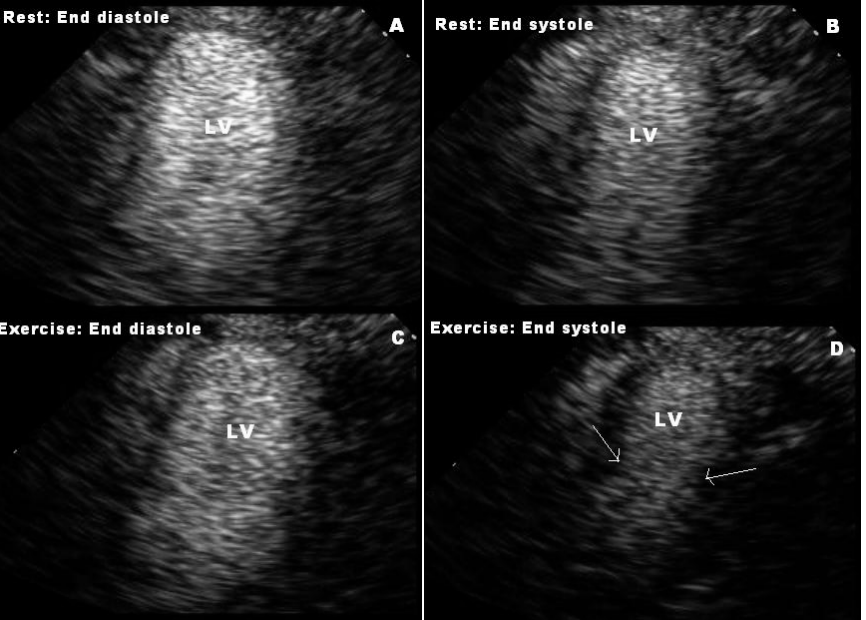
Apical 2-chamber views taken during treadmill exercise testing, demonstrating mid to apical akinesis which develops during stress. Images A and B are taken during rest, and C and D are at peak stress, during diastole (Images A and C) and systole (Images B and D) Image D shows mid to apical akinesis, arrows indicate "hinge point" between basal hyperkinesis and mid to apical akinesis. (LV: Left ventricle).
